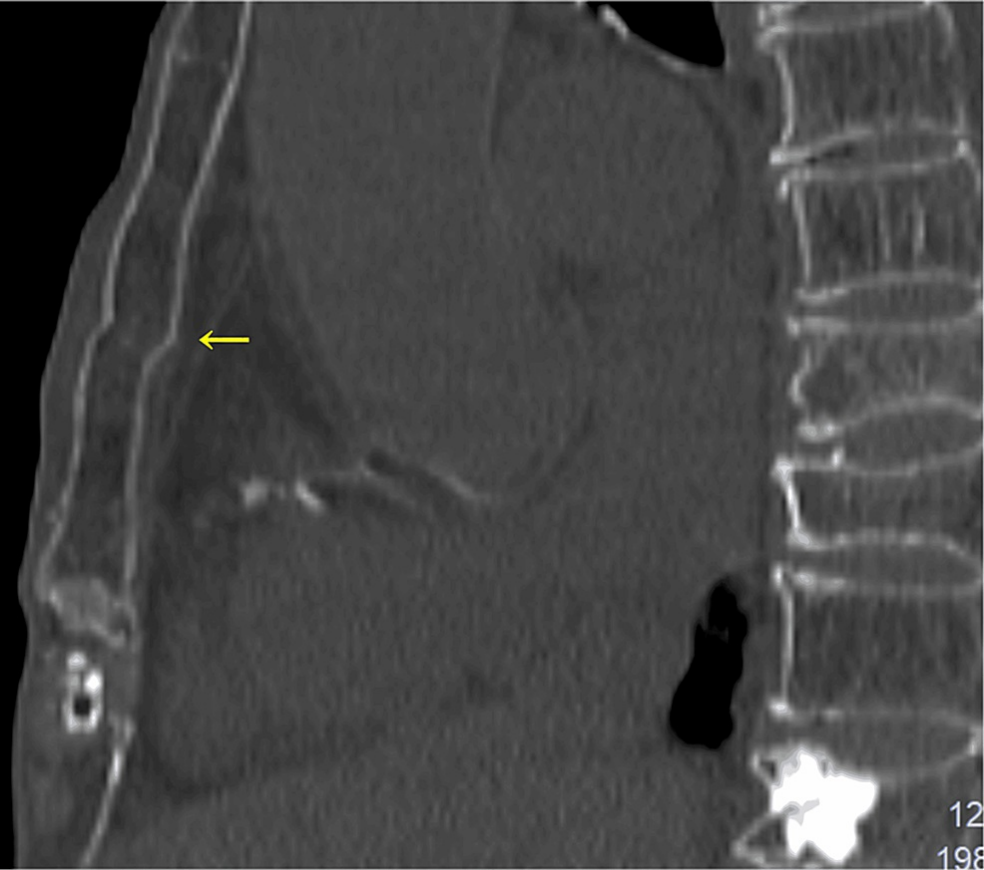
A re-review of computed tomography obtained in the emergency department indicating a sternal fracture (yellow arrow).
